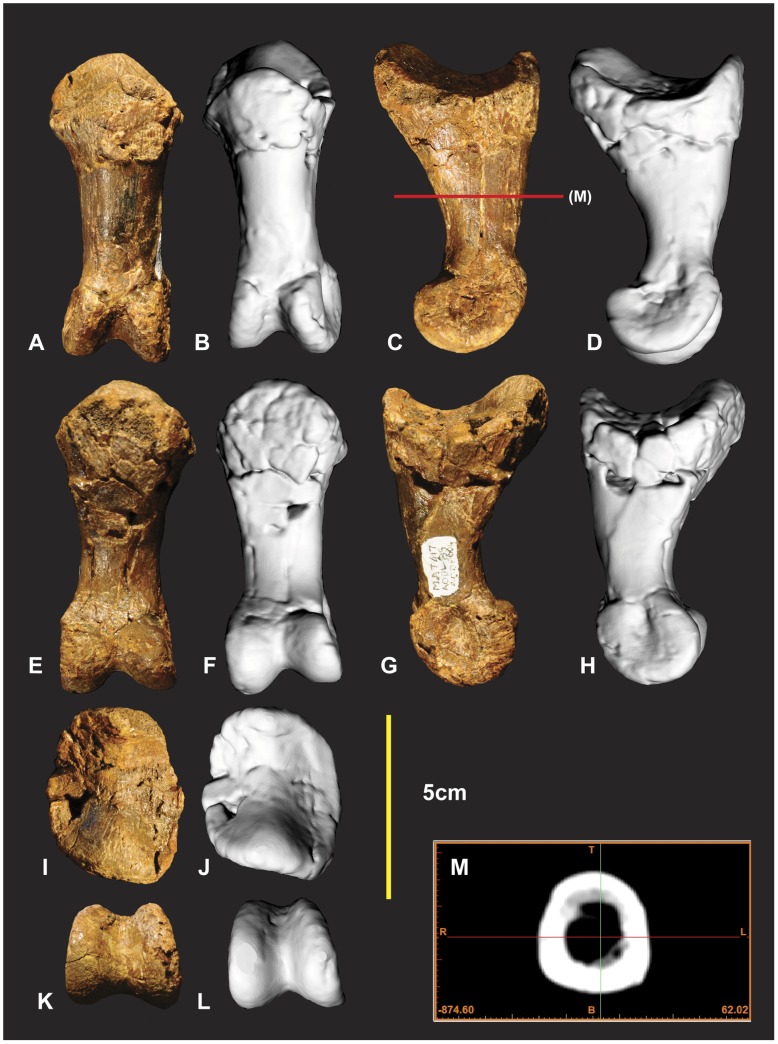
Left manual phalanx II-1. Left manual phalanx II-1 in dorsal (A & B), lateral (C & D), ventral (E & F), medial (G & H), proximal (I & J), distal (K & L), mid shaft CT image (M).
